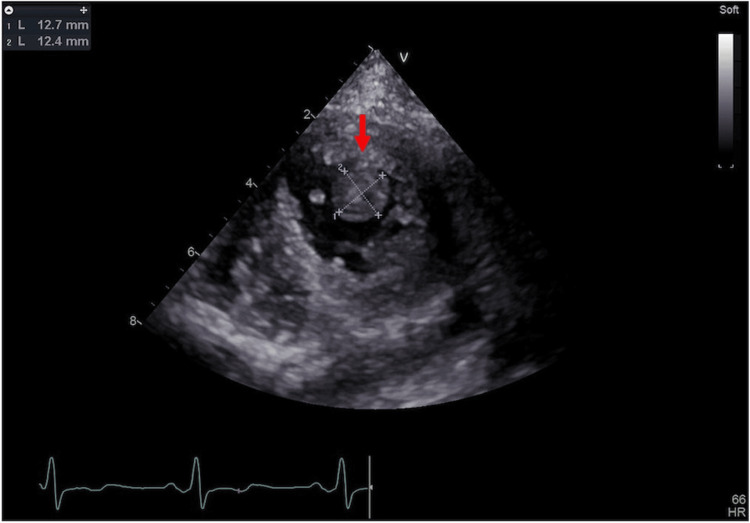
Left ventricular thrombus in the apex (parasternal short axis view apical level) Transthoracic echocardiography on postoperative day 9 showing a 13 mm × 12 mm poorly mobile isoechoic lesion in the apex of the left ventricle (red arrow)
